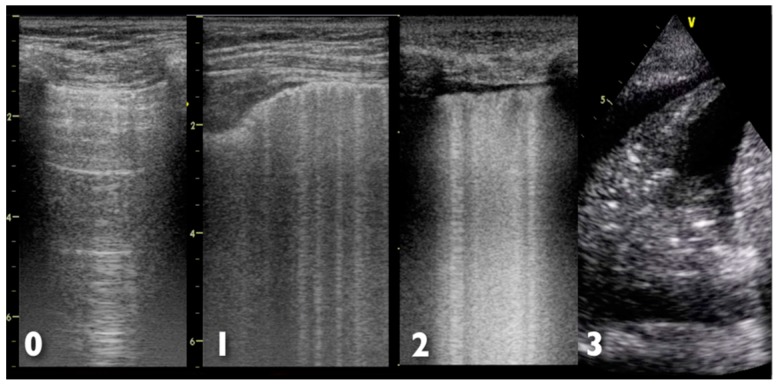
Possible ultrasonographic findings at lung examination. 0: Normal aeration with normal sliding, with A-lines pattern; 1: Multiple B-lines but separated by at least 5 mm; 2: Multiple, coalescent, not well-separated B-lines; 3: Lung consolidation, hyperechoic area with air bronchogram. Numbers on the left side of each ultrasound image represent the depth (in cm).
